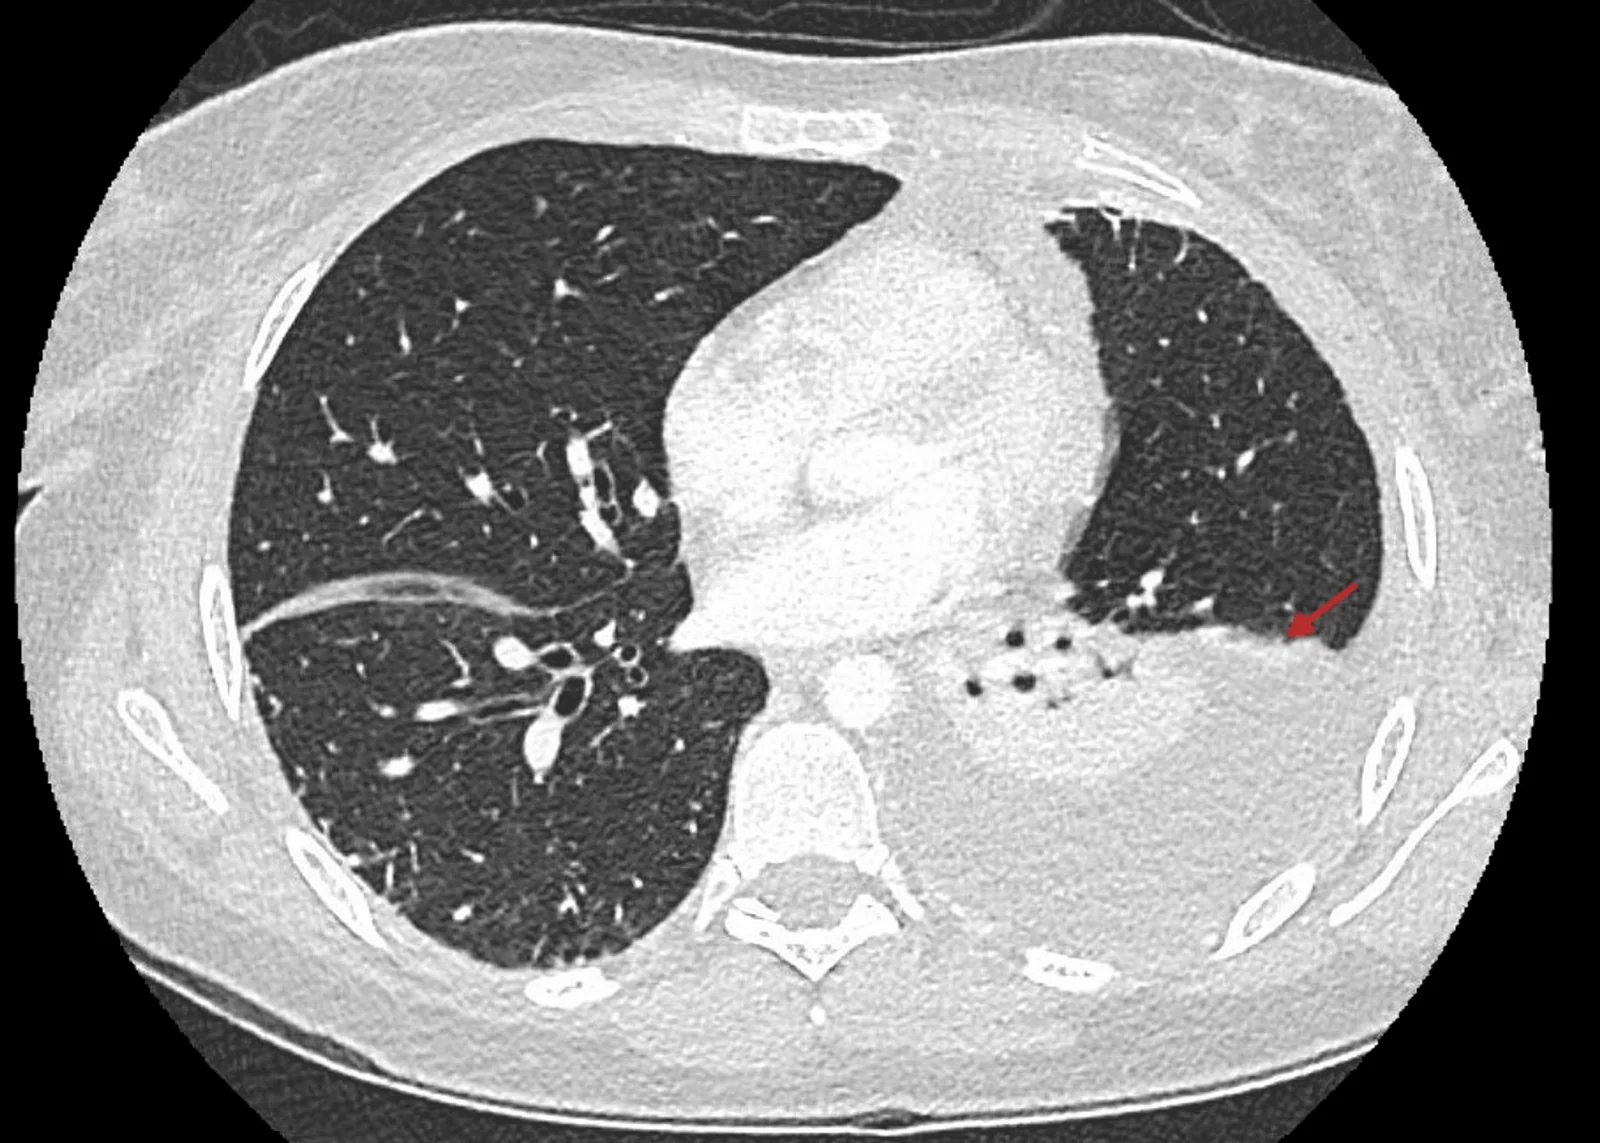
Contrast-enhanced CT of the thorax showing moderate left pleural effusion with underlying lung collapse and air bronchograms secondary to passive compressive atelectasis (red arrow) CT, computed tomography
